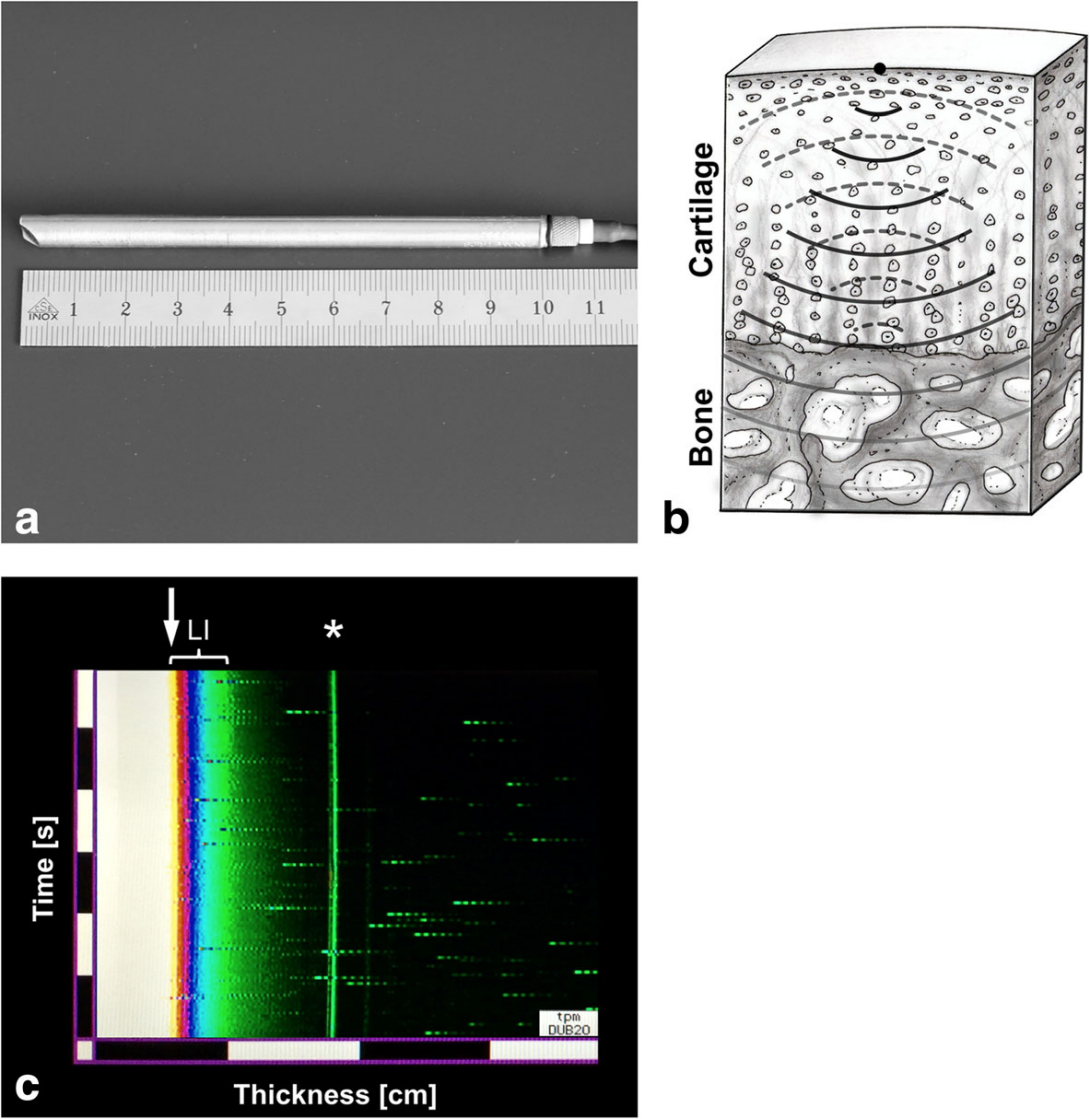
a A pen-like ultrasonic transducer was used (DUB micro®, Rev. 2.14a, Taberna pro medicum, Lüneburg, Germany). b The ultrasonic signal fades while traveling through cartilage. At the cartilage–bone border, the signal is partially reflected (dashed lines). Cartilage thickness is calculated based on the time the reflected signal needs to travel through cartilage. Therefore, thickness directly relates to speed of sound in cartilage. c A-mode ultrasonic image: upper cartilage border (arrow); “leading interface” (LI), an interference pattern which occurs due to the great difference in acoustic impedance of cartilage and gel; cartilage–bone border (asterisk)
